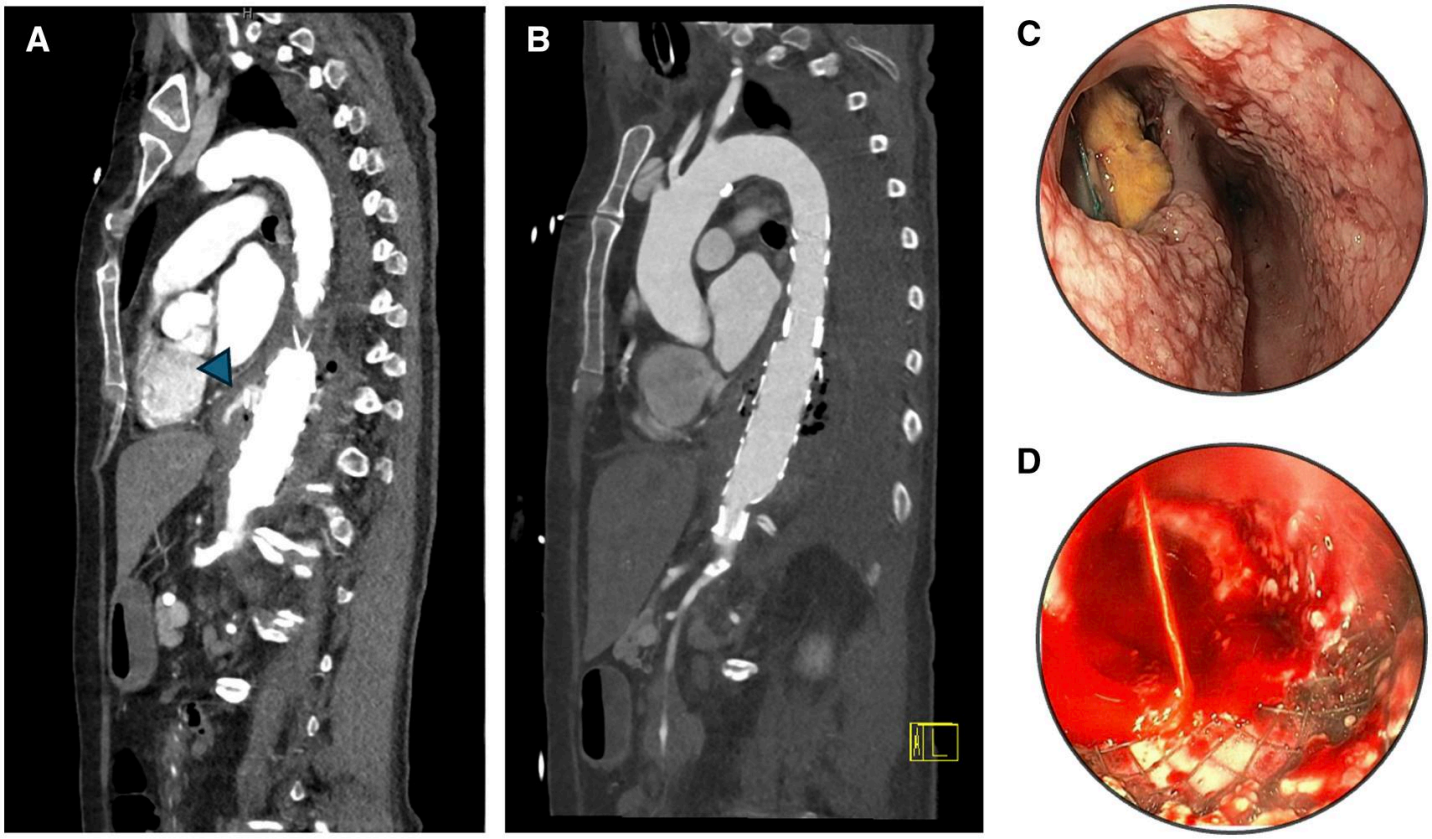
CT Scans and Endoscopy Images of AEF. (A) AEF in patient #4 before and (B) after TEVAR-relining. The arrow shows active bleeding. (C) Esophagoscopy of patient #4 after TEVAR-relining. The aortic stent is clearly visible in the mediastinum. (D) Endoscopic image of the oesophagus of patient #2 with oesophageal stent *in situ*. Abbreviations: AEF, aorto-oesophageal fistula; TEVAR, thoracic endovascular aortic repair
